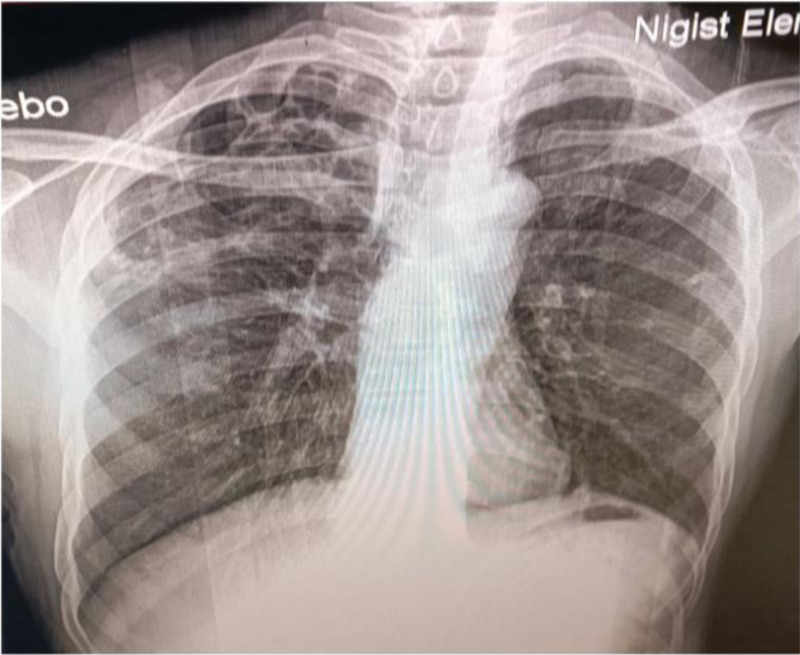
A chest x-ray shows right upper lung zone fibrotic change with no evidence of septic emboli to lung.
